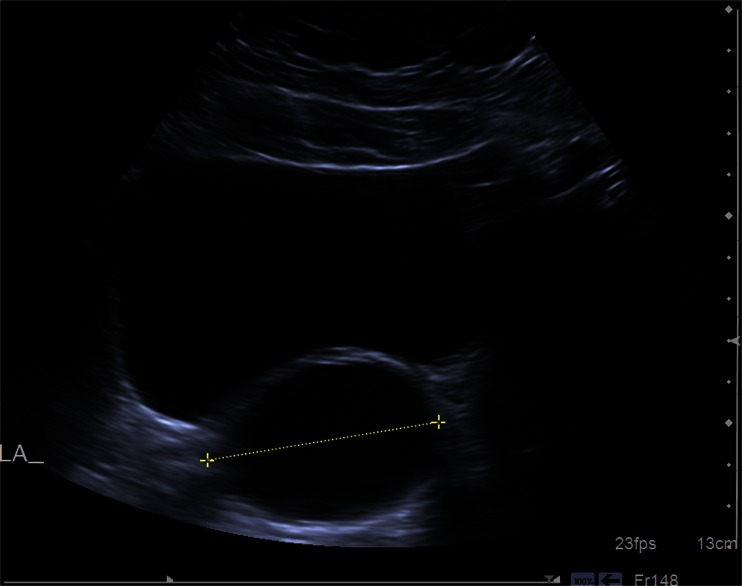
Follow-up ultrasound image (shows pathology)
